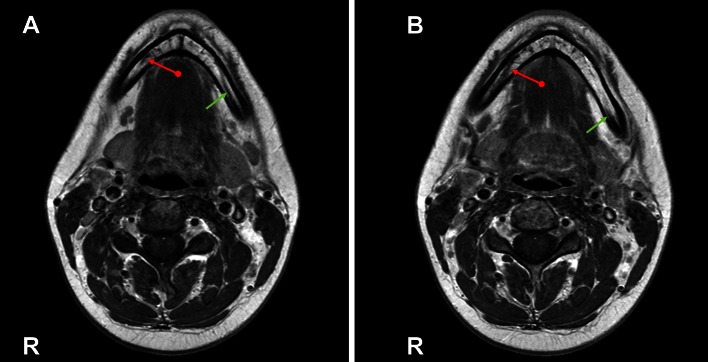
Axial 3T-MRI images (T1w turbo spin-echo sequence with contrast agent) showing neuritis of the right IAN at molar and premolar levels (*red arrow* with *round end*, b). The neuritis can be identified by the asymmetrical thicker appearance of the IAN at the *right side* (b), compared to the normal left IAN (a) (*green arrow*, a)
